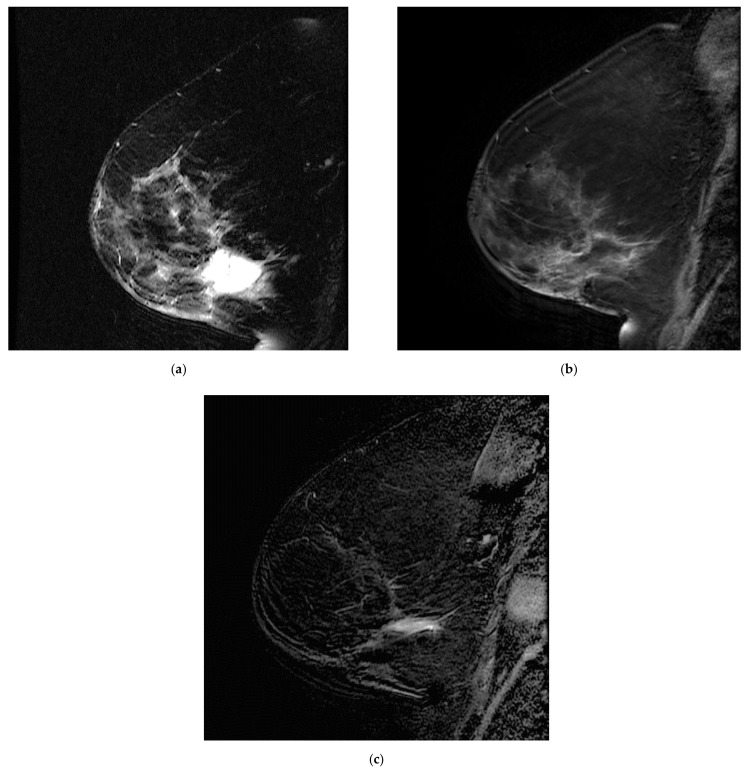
Postoperative seroma with surrounding non-mass enhancement on MRA (a) T2-weighted sequence and (b) T1 fat sat with gadolinium. The seroma resolved and linear enhancement is seen in the same location on (c) MR B. This was initially attributed to post-surgical changes and later confirmed to correspond to disease recurrence.
